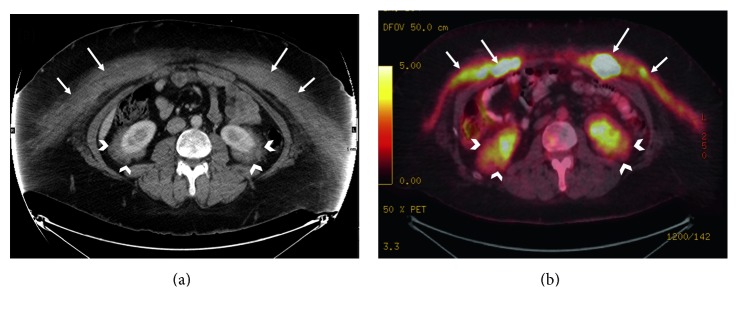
(a) Axial contrast-enhanced CT image of the abdomen and pelvis at the level of the kidneys shows bilateral rind-like perirenal soft tissue infiltrations, giving the appearance of “hairy kidney” (arrowheads). Anterior abdominal wall infiltration is also seen (arrows). (b) Corresponding PET/CT image shows intense FDG uptake in the same areas.
